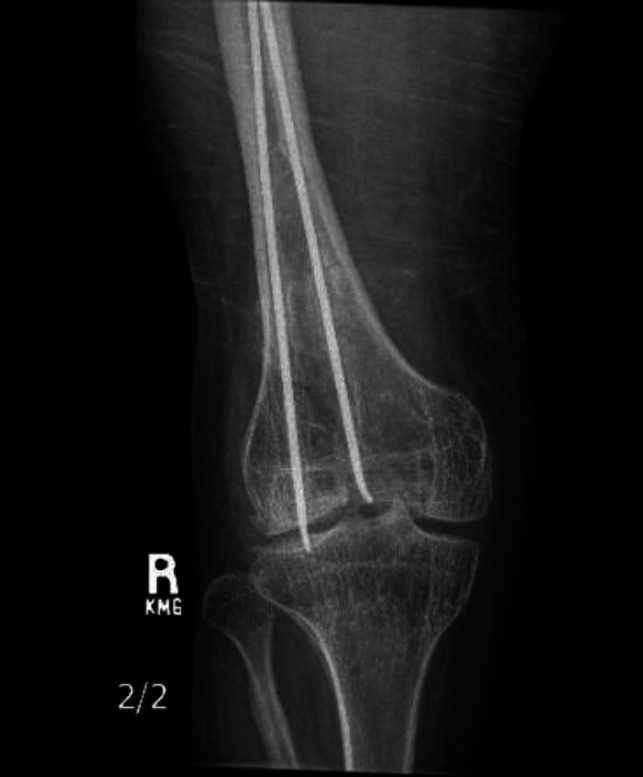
AP radiograph showing the right distal femur of a 30-year-old male patient with retained flexible nails that have migrated into the knee.
